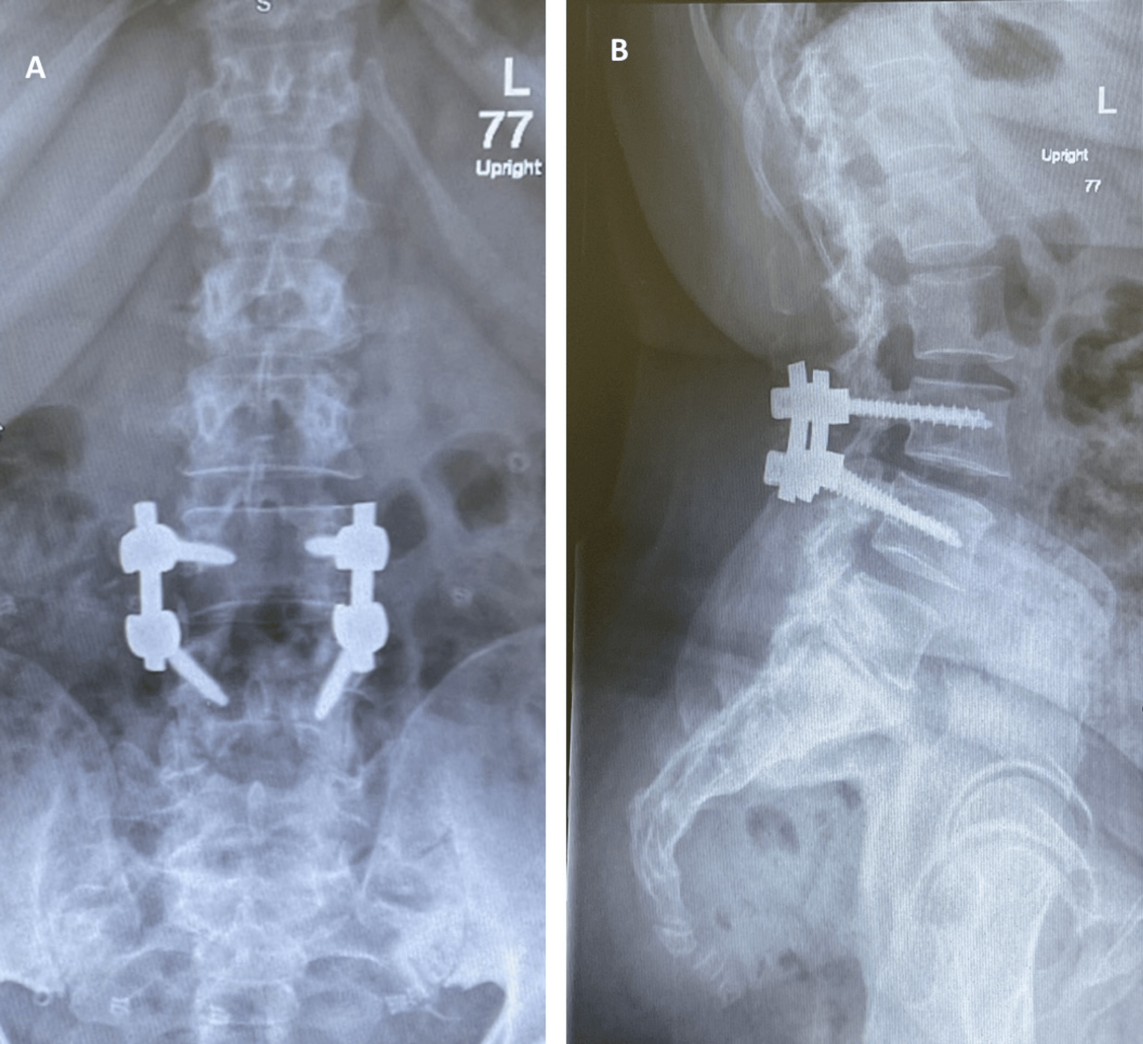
Postoperative lumbar X-ray Anteroposterior (AP) view (A) and lateral view (B) show no significant changes since the previous study.
